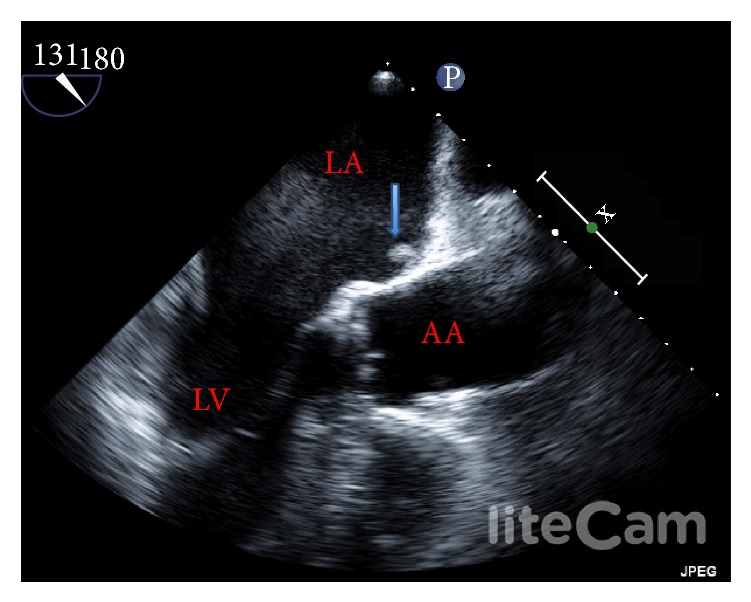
Transesophageal echocardiogram showing a thrombus in the left atrium (arrow). LA: left atrium, LV: left ventricle, and AA: ascending aorta.
